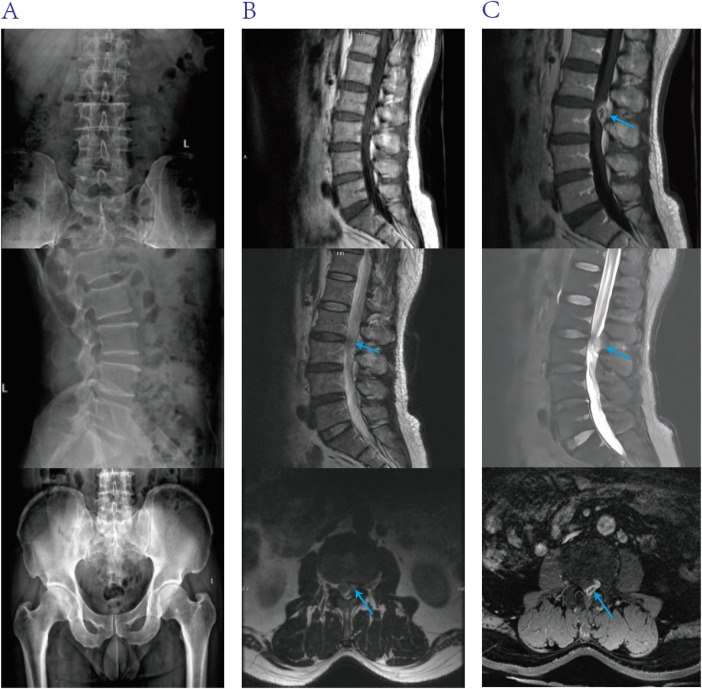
Preoperative radiographic and MRI evaluation. (A) Standing anteroposterior and lateral radiographs of the lumbar spine, along with an anteroposterior pelvic radiograph, showing degenerative changes without obvious structural abnormalities. (B) Non-contrast MRI sequences. From top to bottom: sagittal T1-weighted image, sagittal T2-weighted image, and axial T1-weighted image. A posteriorly migrated extradural lesion at the L2/3 level is indicated by blue arrows. (C) Contrast-enhanced MRI sequences. From top to bottom: sagittal T1-weighted image with gadolinium enhancement, sagittal T2-weighted image, and axial post-contrast T1-weighted image. The lesion demonstrates irregular peripheral enhancement and causes rightward displacement of the dural sac (blue arrows).
